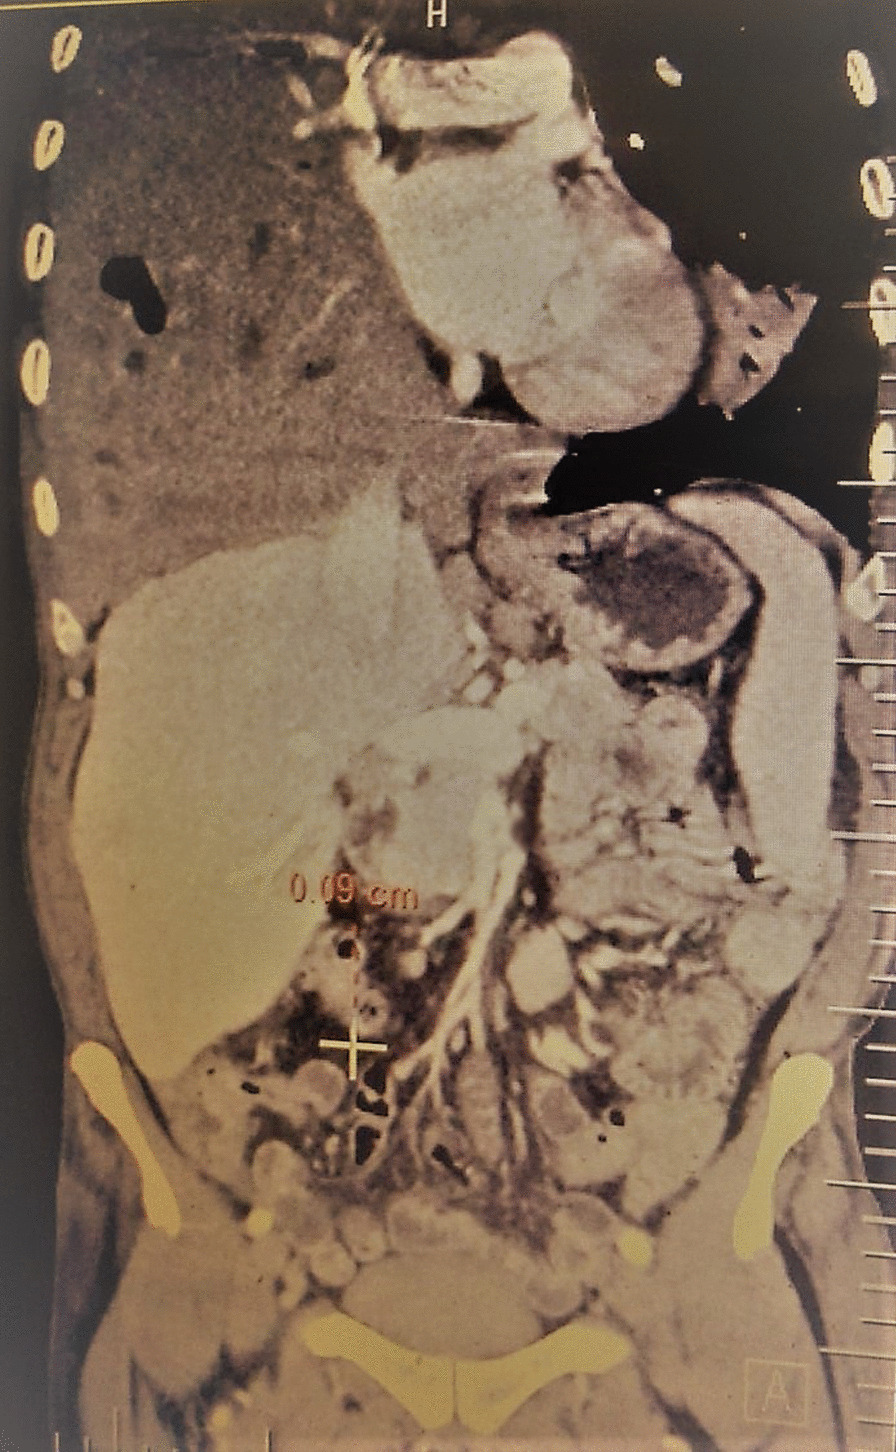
Computed tomography chest and abdomen (coronal reformatted view) displaying a mass-like dense consolidation of the right lung and an ill-defined heterogeneously hypodense lesion at the caudate lobe of the liver suggestive of liver metastasis
